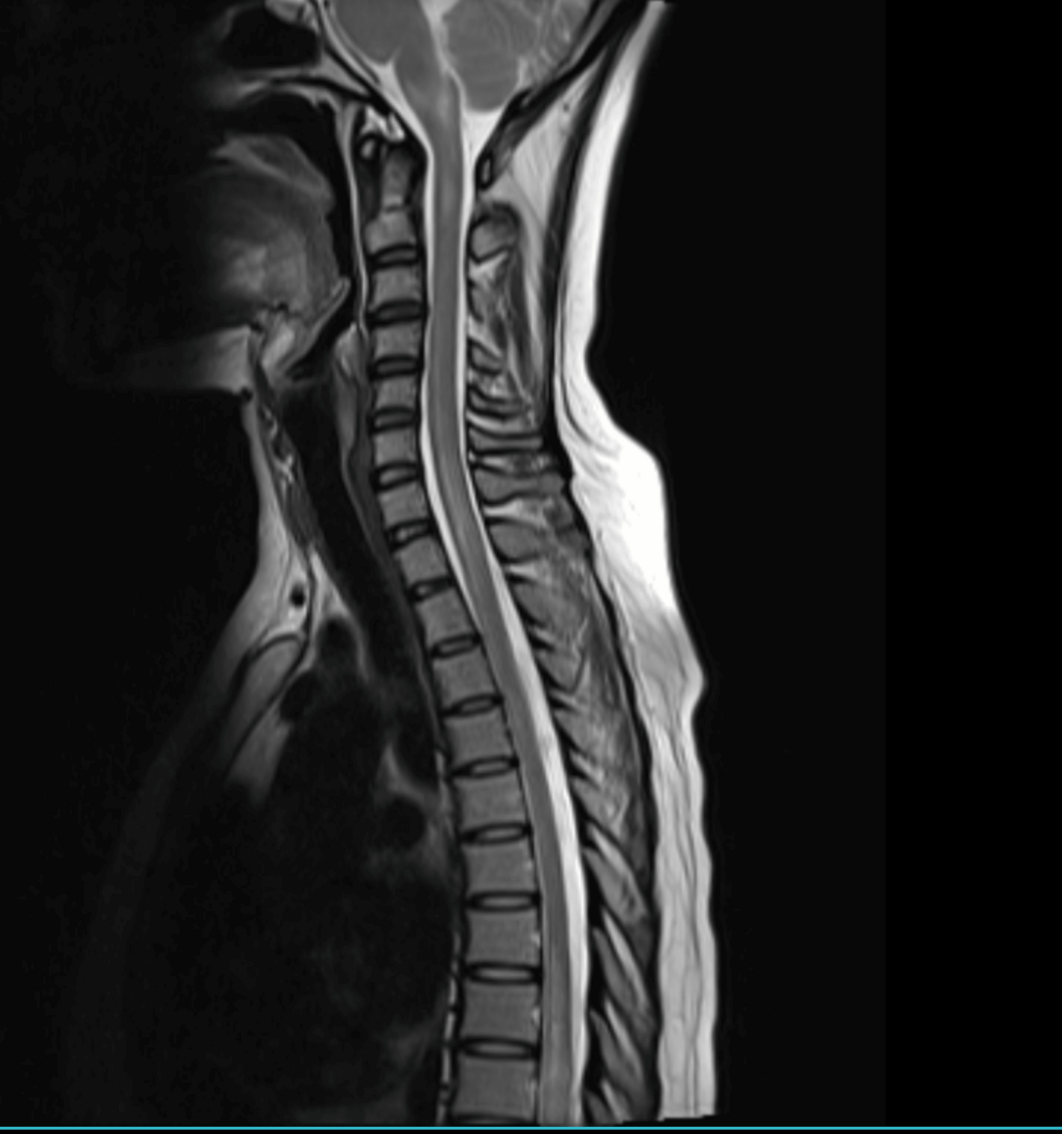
T2-weighted MRI of the spine showing LETM extending from the cervico-medullary junction to the D9 spinal level LETM, longitudinally extensive transverse myelitis
